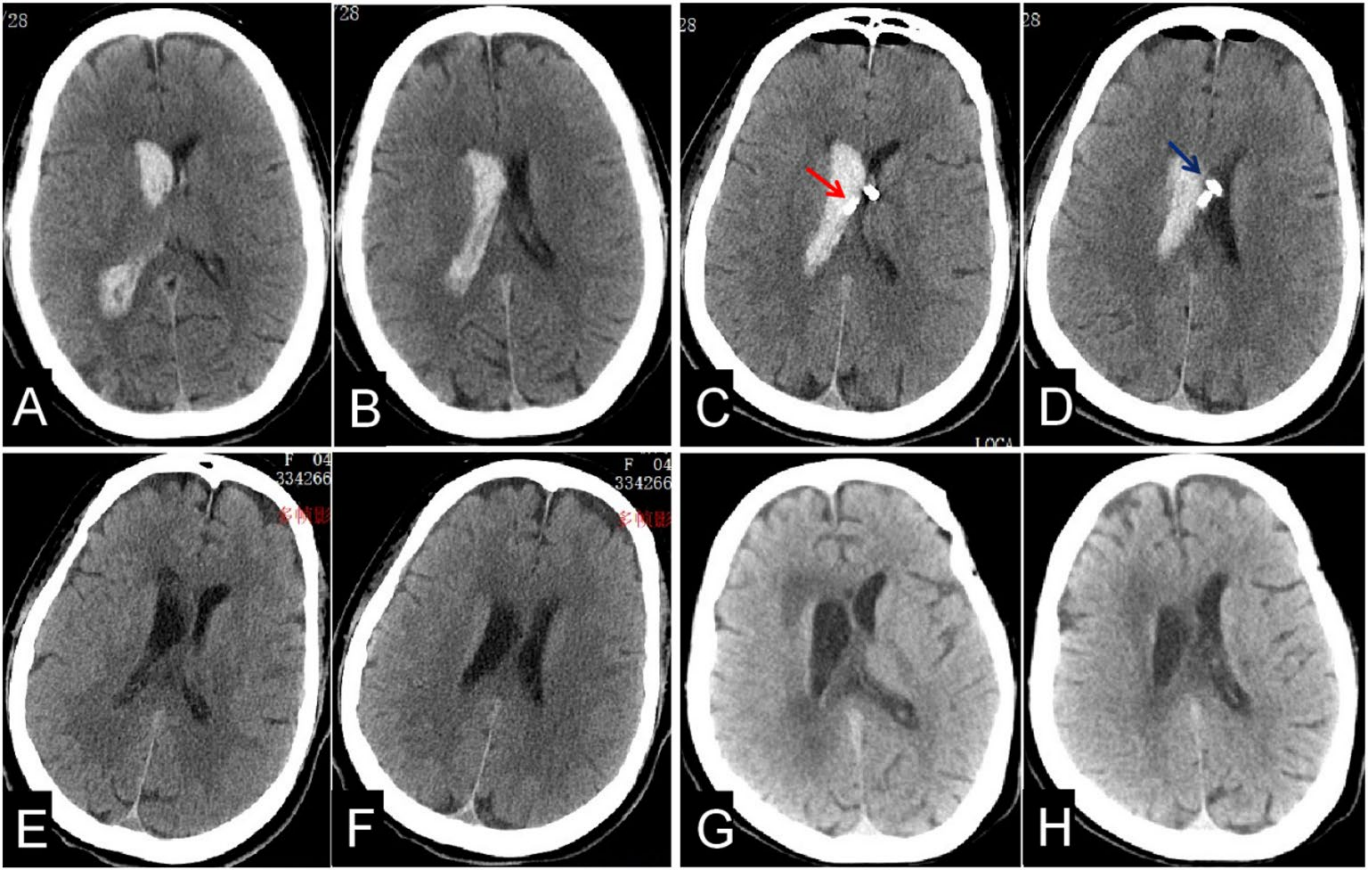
The sub-optimal placement of EVD catheter was shown by the CT scan. IVH was shown at 3 h pre-operation (A,B); Both EVD catheters were shown in the contralateral ventricle at 1 d post-operation by freehand (the left catheter shown by the red arrow and the right one shown by the blue one, (C,D); The catheters were removed and IVH was completely cleared at 2 w after EVD (E,F); The right ventricle was enlarged at 1 m follow-up (G,H).
